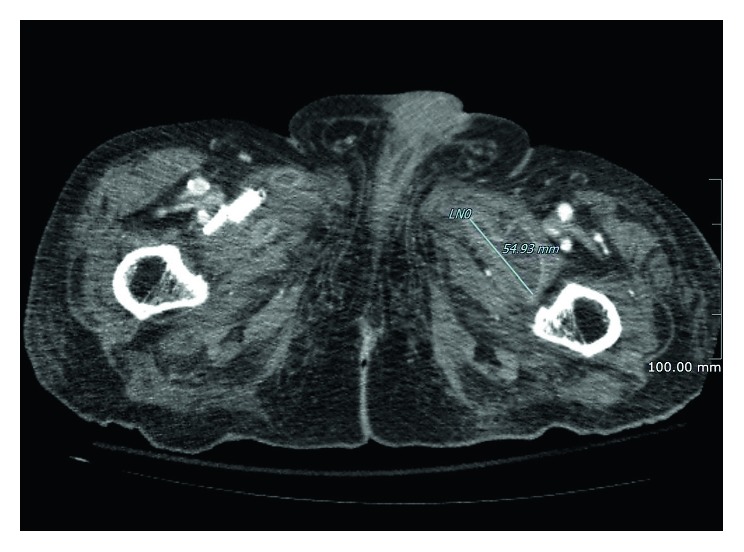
Transverse section of the same CT scan showing the left collection yet a drastic dimensional reduction of the right collection.
